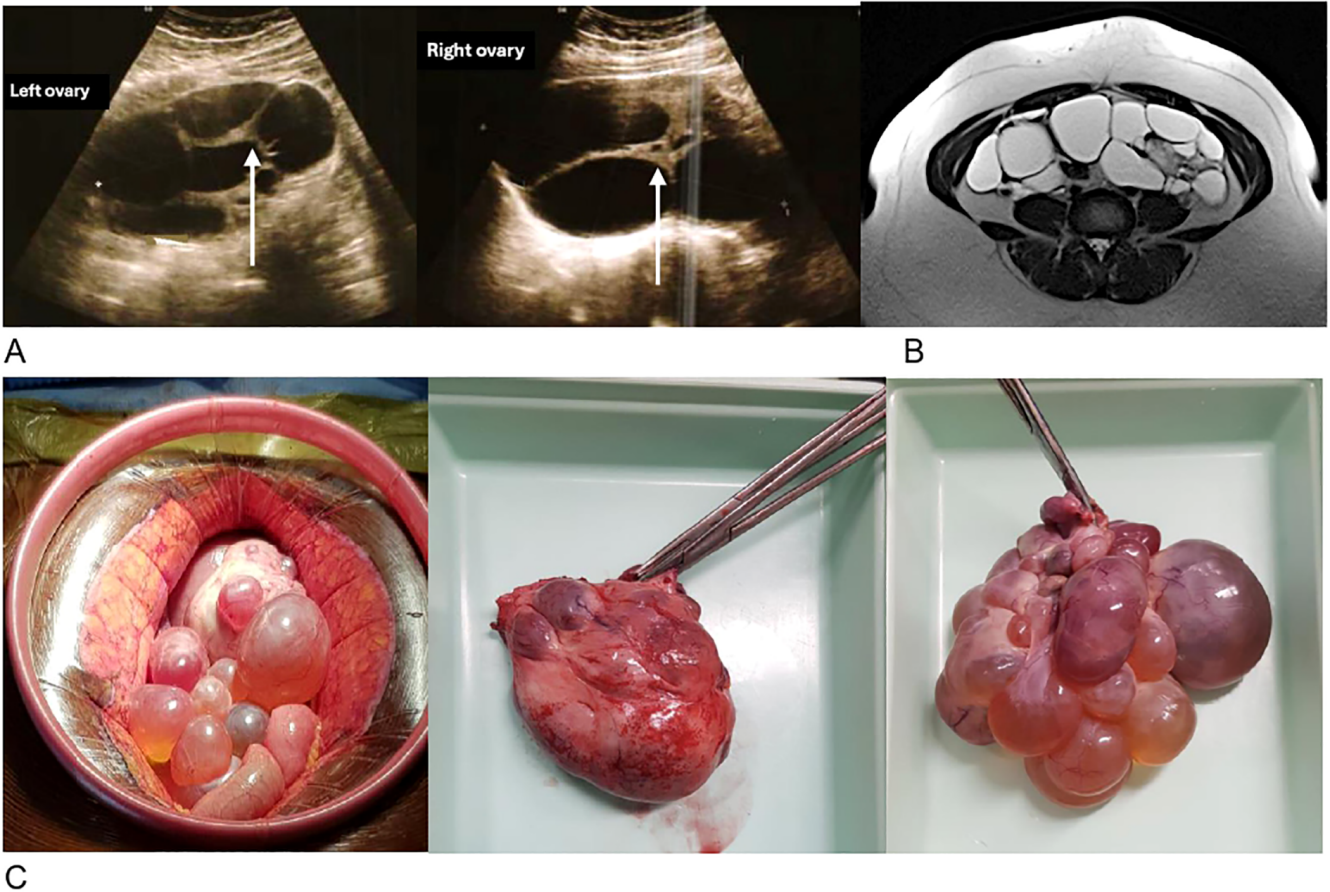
Morphological radiological and surgical appearance of the ovaries. (A) Transvaginal ultrasound of multicystic ovaries with thick walls (arrow). (B) Axial section of the pelvic MRI in T2 showing bilateral ovarian cysts. (C) Pre- and post-operative photographs of the left ovary weighing 268g (left) and the right ovary weighing 390g (right).
